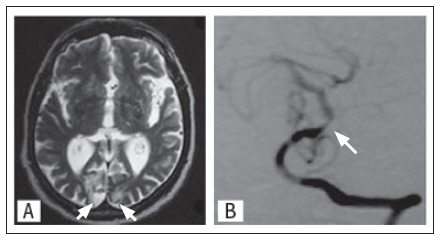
A. T2-weighted magnetic resonance imaging from Case 1, showing bilateral occipital infarcts (arrows). B. Digital subtraction angiography showing severe basilar artery stenosis (arrow).
